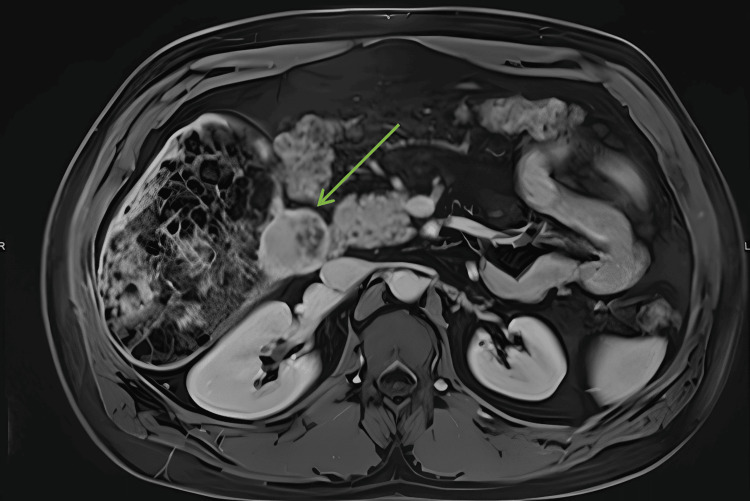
Repeat contrast-enhanced MRI of the liver Growth of mural nodules, especially the medial lesion adjacent to the head of the pancreas (shown in green arrow) MRI: magnetic resonance imaging
